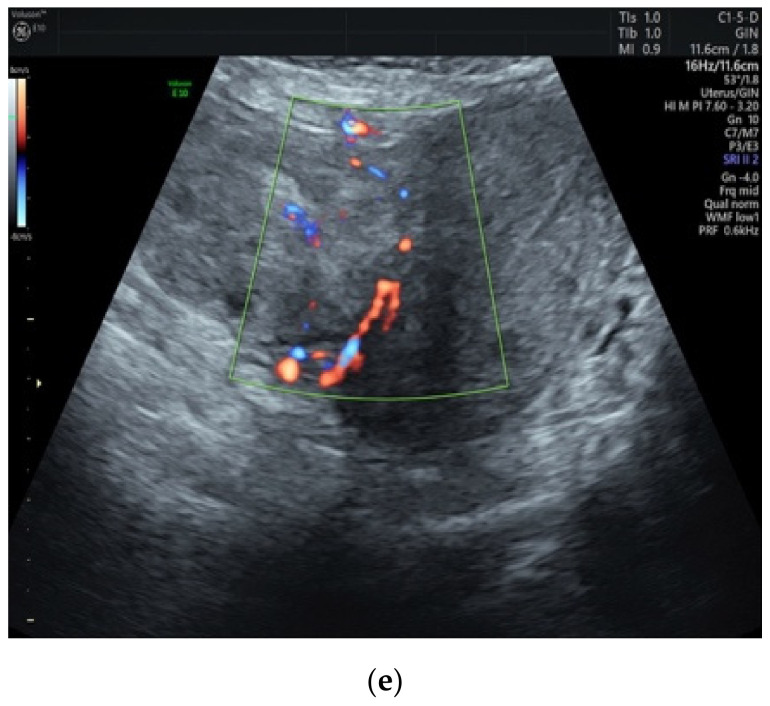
Uterine cotyledonoid dissecting leiomyoma and ultrasound features. Different US features of uterine cotyledonoid dissecting leiomyoma: note in (a) irregular margins and heterogeneous aspect of the mass; no evidence of capsule, no shadowing; (b) hypervascularization compared with the vascularity of the myometrium, color score 3; (c,d) the presence of colliquated areas; (e) note the uterus on the right side and the lesion on the left side emerging from the lateral wall of the uterine corpus. With the application of color doppler, the vascularization is visualized with color red and blue, in images (b–e).
